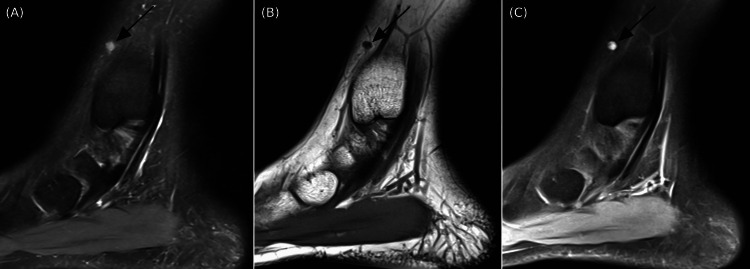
Ankle MRI sagittal images of a small lesion in the subcutaneous fat just below the skin surface at the lateral ankle measuring 3x4x5 mm A: Lesion is hyperintense (black arrow); proton density fat saturation MRI sequence. B: Lesion is hypointense (black arrow); T1-weighted MRI sequence. C: Lesion is avidly enhancing (black arrow); T1-weighted fat saturation MRI sequence with gadolinium contrast.
